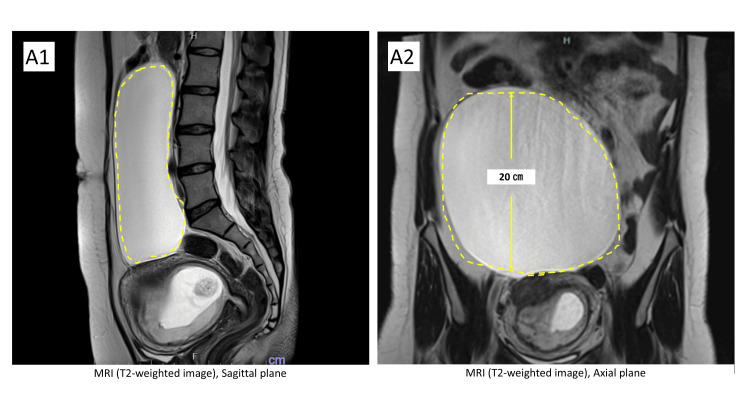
MRI at 11 weeks’ gestation A1.2: The initial MRI image shows a cyst measuring 20 cm in length located above the uterus.
